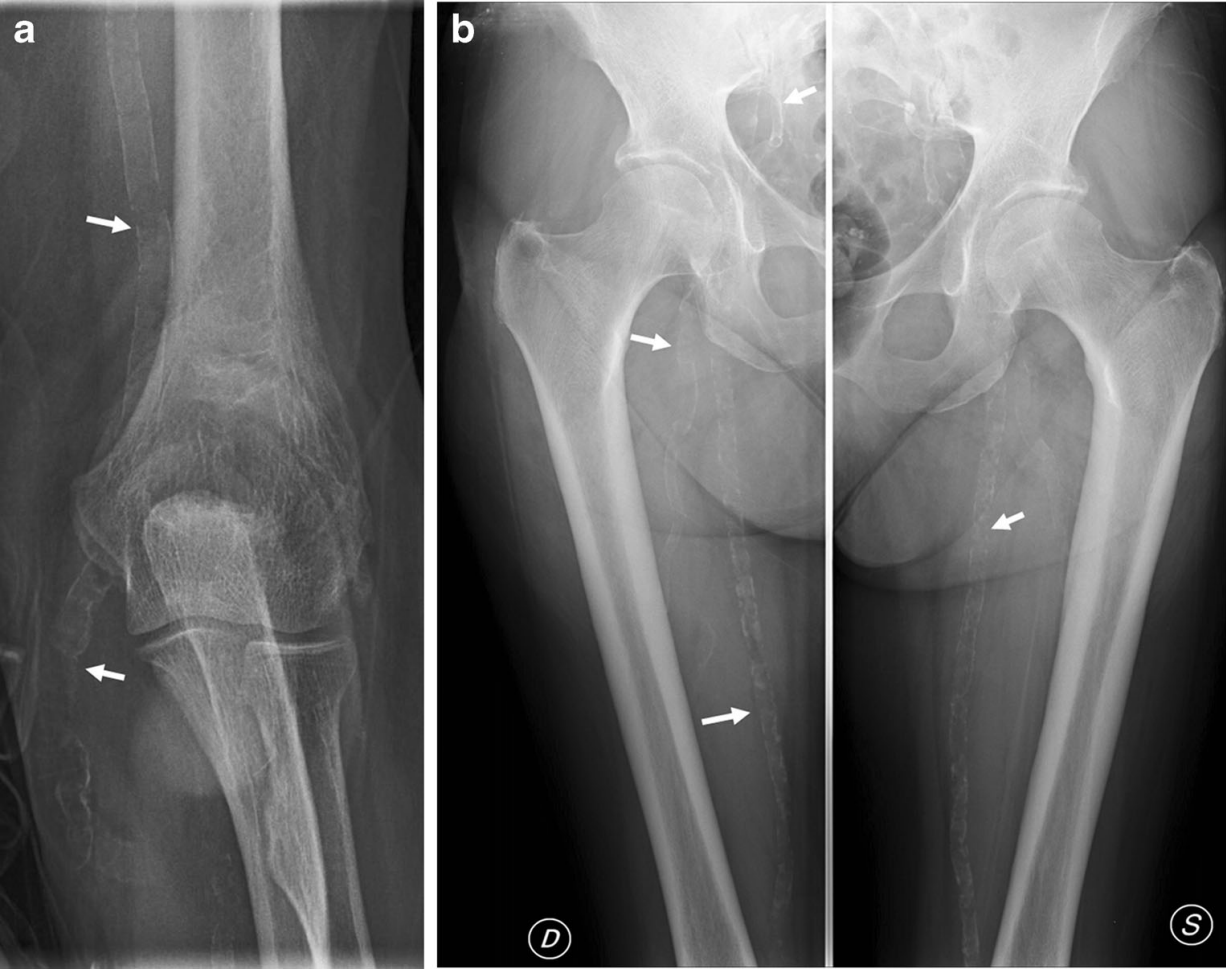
a, b: Radiographic images of several skeletal segments of different patients (all undergoing long-term haemodialysis) show diffuse calcifications of the peripheral arteries (*arrows*). Vascular calcifications appear as both medial and intimal calcifications of arteries
